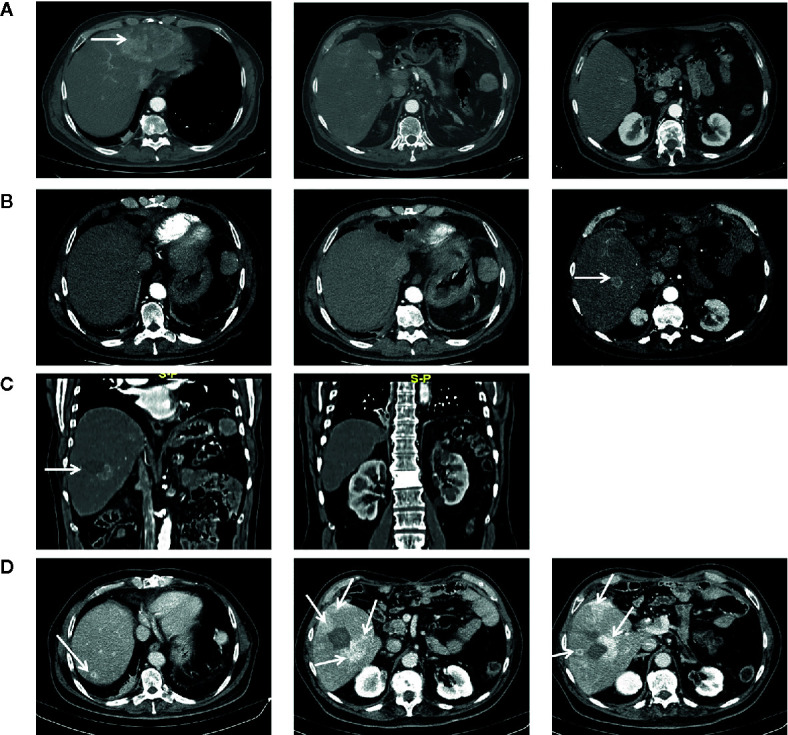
Chest CT-scan performed at diagnosis in March 2017 (A), in October 2017 following first relapse (B), in February 2018 at tumor progression following first percutaneous thermal ablation (C), in May 2018 at tumor progression following second percutaneous thermal ablation and before starting chemotherapy with cisplatin and gemcitabine (D). Arrows indicate tumor lesion.
